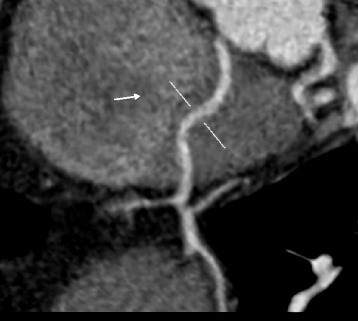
Multiplanar reconstruction computed tomography image demonstrates the intramuscular course of the accessory coronary artery (*arrow*)
